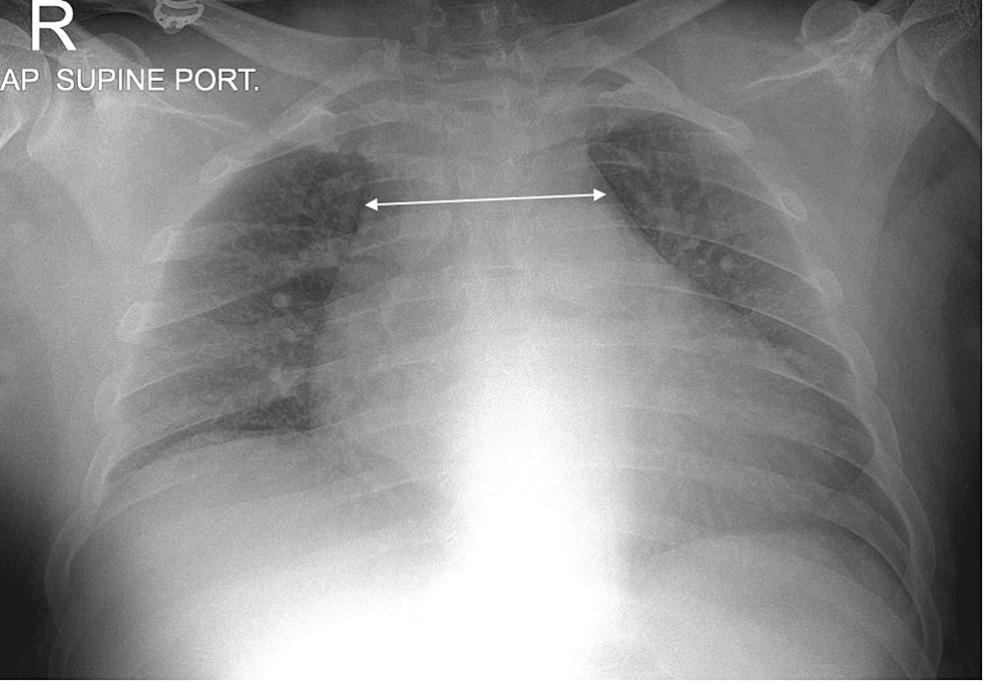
Chest X-ray with prominent mediastinum
